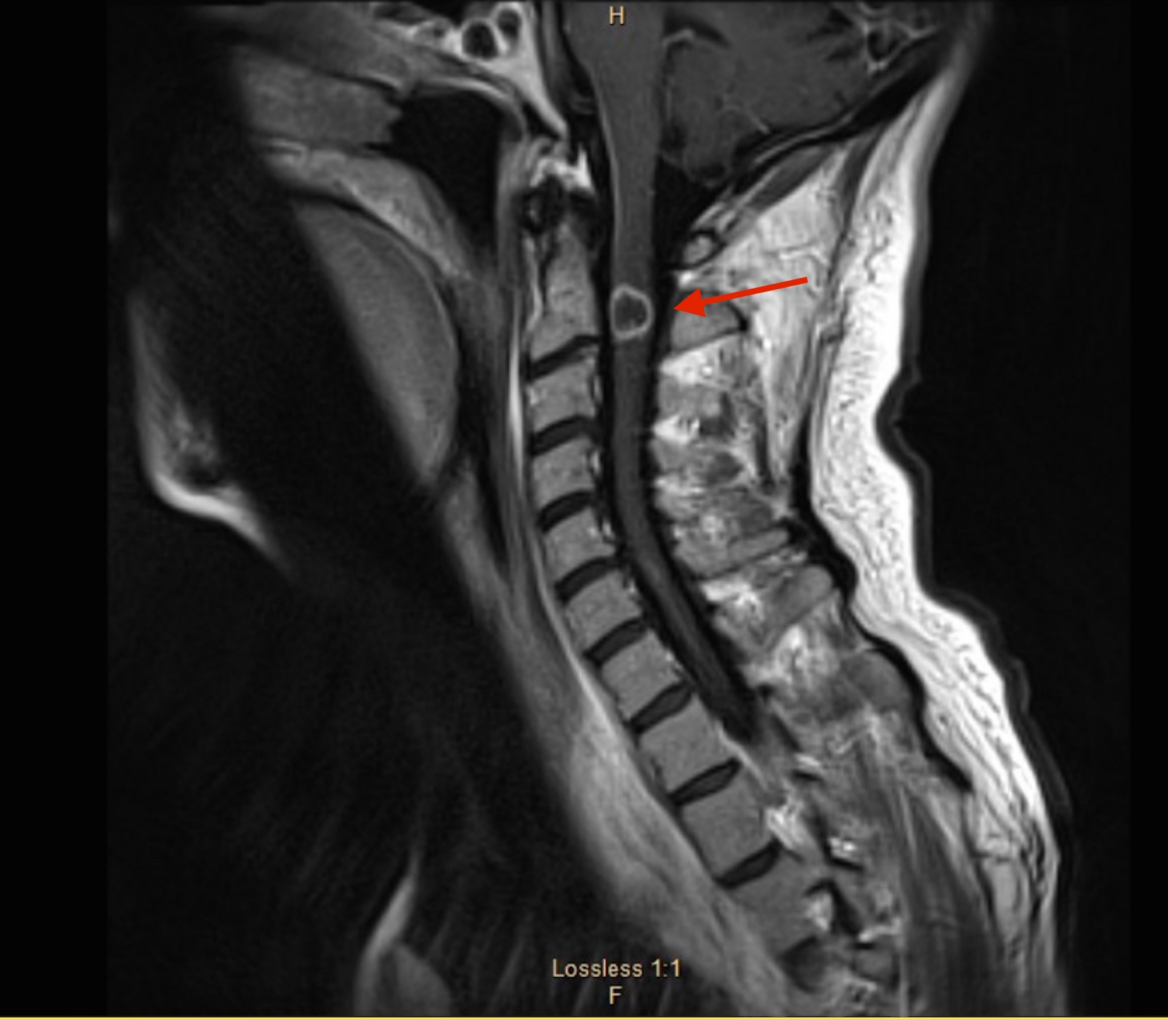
Magnetic resonance imaging (MRI) of the cervical spine showing solitary ring enhancing lesion at C2 (red arrow).
